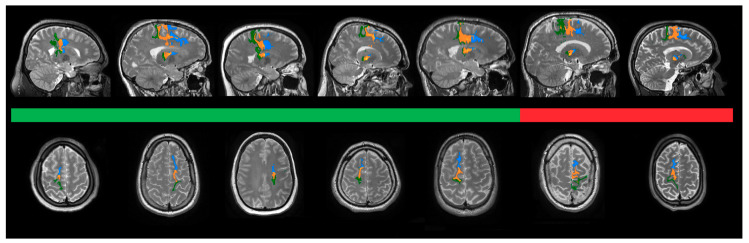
Connectivity-based segmentation of the seed areas. Representative tractographies of each patient. Images are shown in patient-space, where the most significant density of fibers was observed. Therefore, the most symbolic MRI cut of each patient was depicted, including the most representative hemisphere in each individual. Fibers originated from the VTAs that connect to M1 are shown in green, whereas fibers connecting to SMA and preSMA are shown in orange and blue, respectively. Patients are separated in responders (green bar) and non-responders (red bar) in descending order from the best to the worst clinical outcome from left to right. Independently from their clinical outcome, all patients showed thalamic connectivity to the seed areas.
